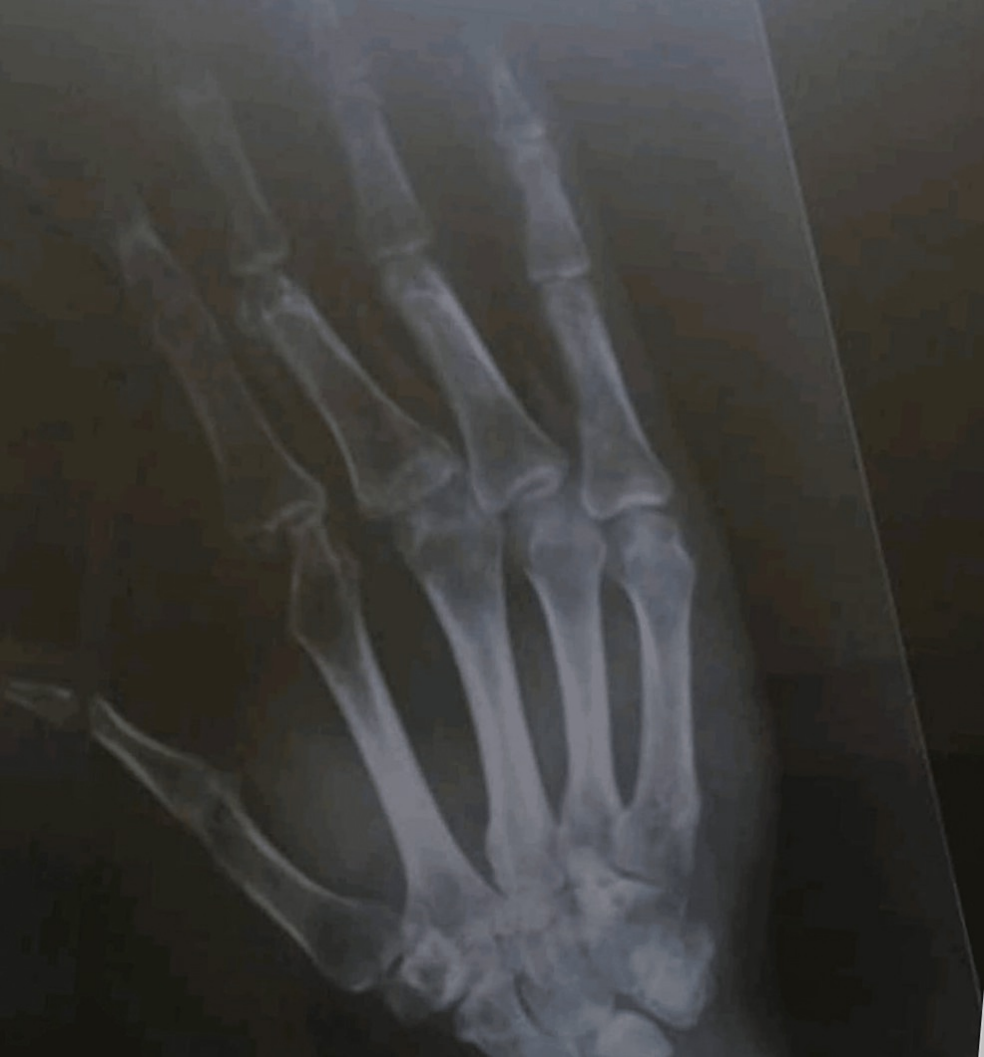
Non-digital radiograph of the hand showing preserved bone grafts at 11 months post-reconstruction
